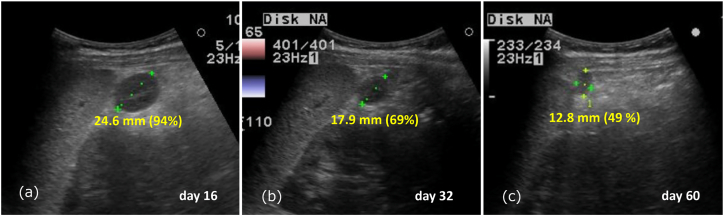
Ultrasound follow-up (a) The accessory spleen shrunk to 24.6 mm (94 %) and blood flow signals ceased at 16 days after the first visit. (b) The accessory spleen shrunk to 17.9 mm (96 %) at 32 days after the first visit. (c) The accessory spleen shrunk to 12.8 mm (49 %) at 60 days after the first visit.
